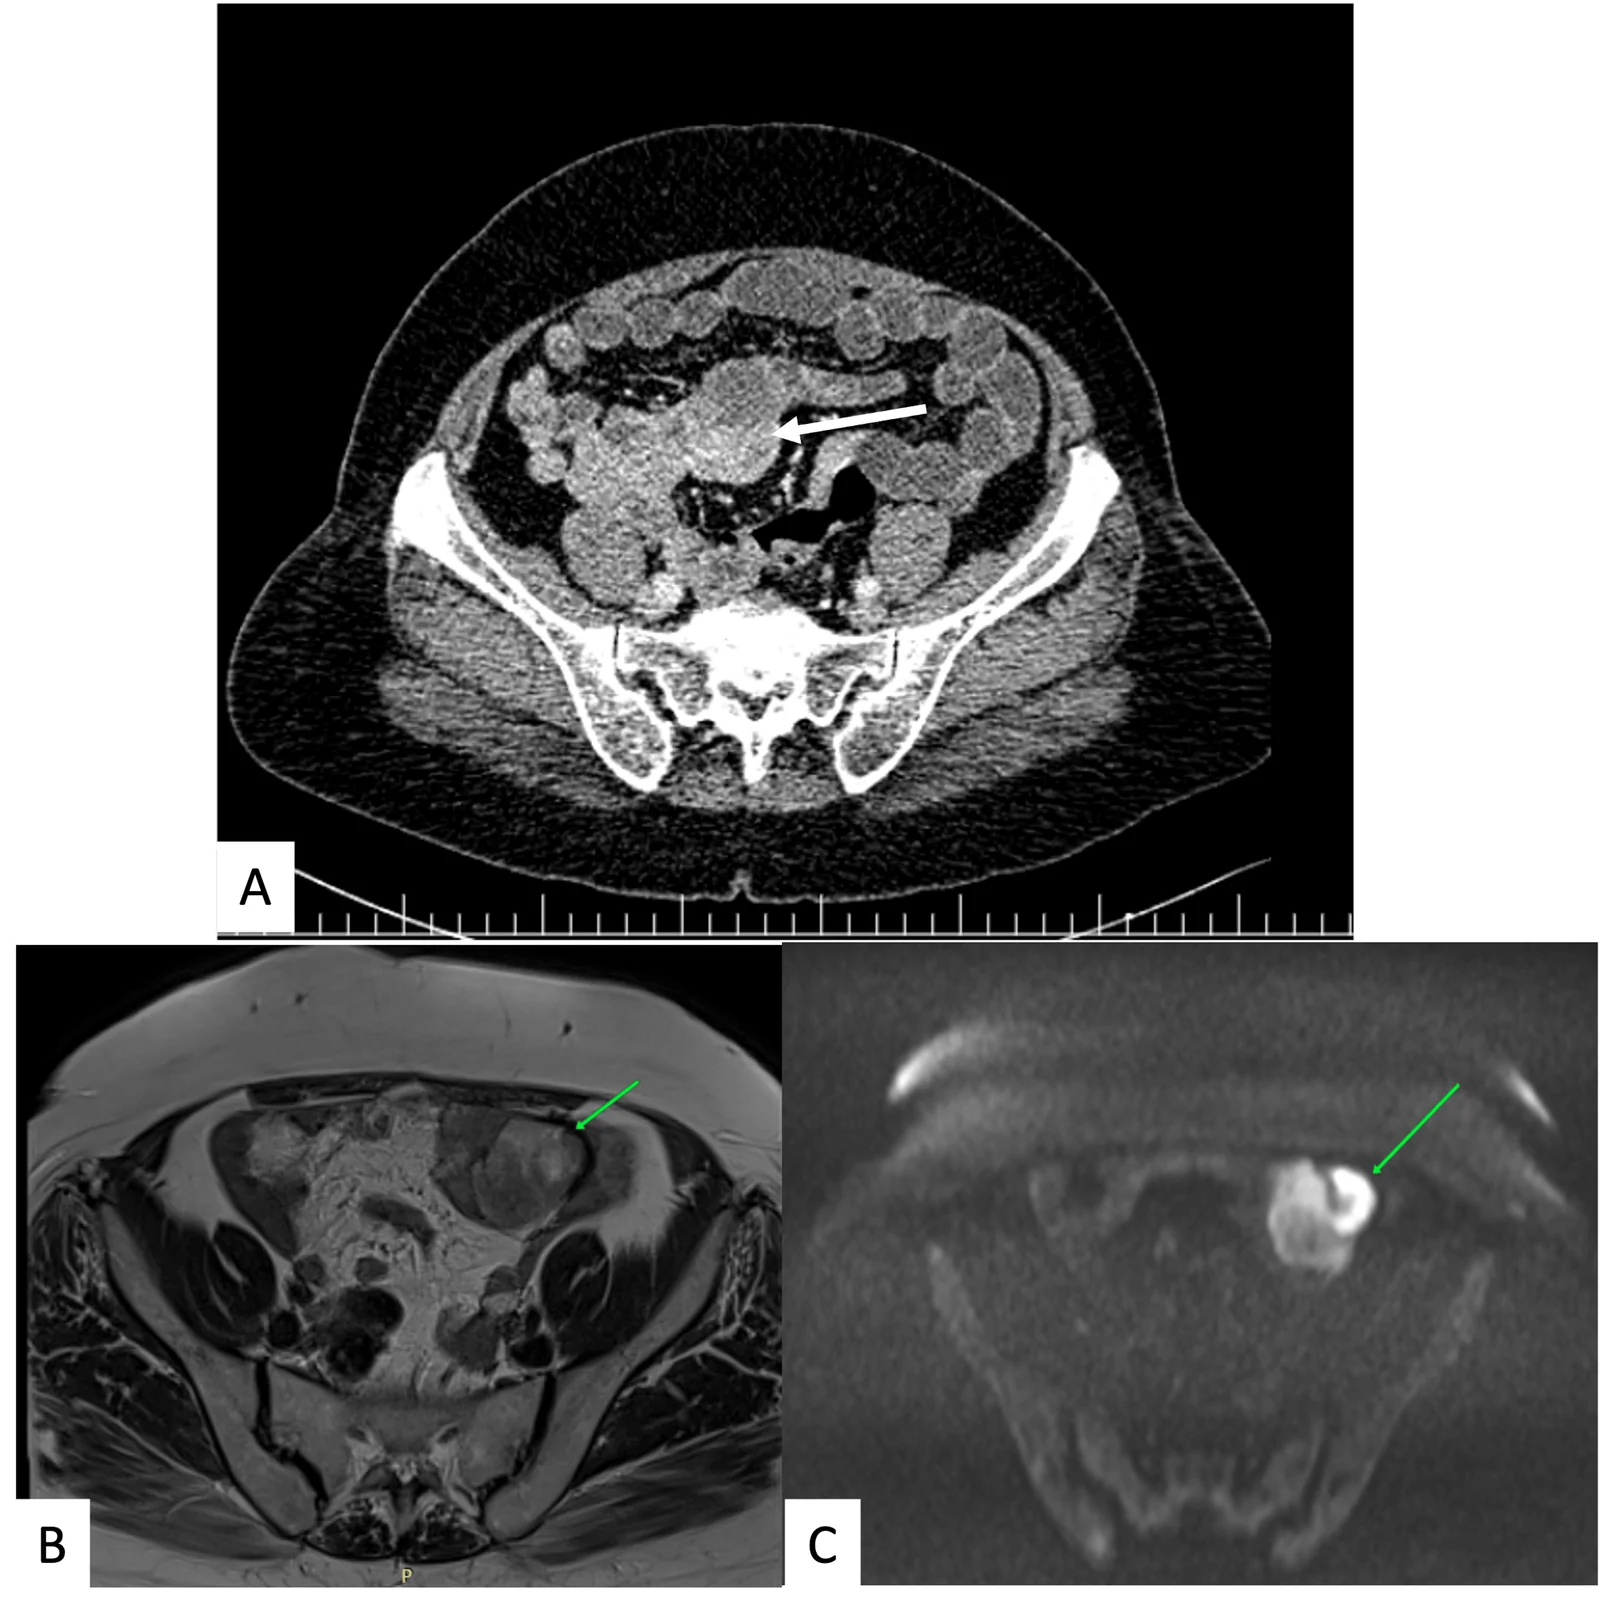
Imaging findings (A) Axial contrast-enhanced abdominal CT scan showing a 4.5-cm exophytic ileal mass with heterogeneous enhancement and a cystic component (white arrow), without lymphadenopathy or ascites. (B) Axial T2-weighted pelvic MRI demonstrating a well-defined ileal mass in the right iliac fossa with intermediate T2 signal intensity (green arrow). (C) Axial diffusion-weighted MRI showing high signal intensity of the lesion, suggesting restricted diffusion (green arrow), consistent with a gastrointestinal stromal tumor CT: computed tomography; MRI: magnetic resonance imaging
